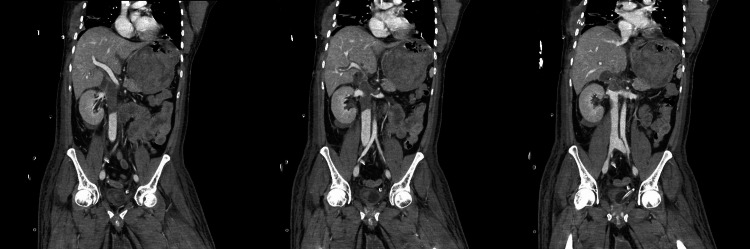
Multiple cuts of a CT abdomen showing a large thrombus of the distal IVC sparing the renal vessels and a blood-filled stomach. IVC: Inferior vena cava
